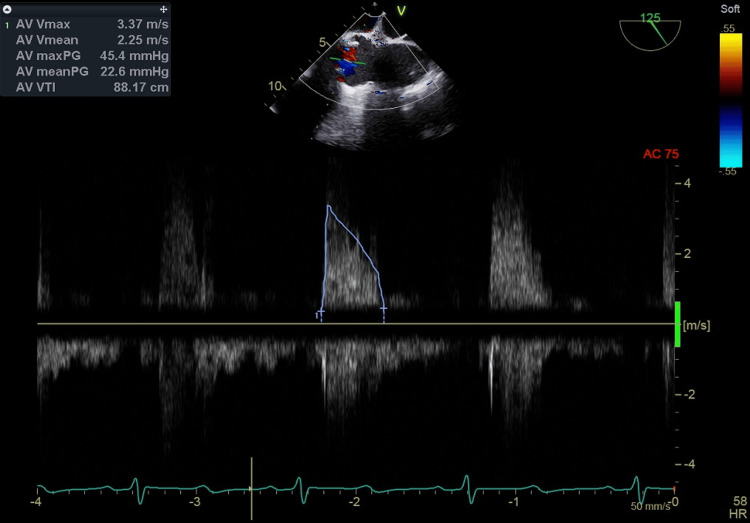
Assessment of blood flow velocity in accelerated blood flow corresponding to moderate AS by continuous wave Doppler of TEE after CPB It revealed a maximum aortic valve blood flow velocity of 3.4 m/s, a maximum aortic valve pressure gradient of 45 mmHg, and a mean aortic valve pressure gradient of 23 mmHg, indicating findings corresponding to moderate AS. AS: aortic stenosis; TEE: transesophageal echocardiography; CPB: cardiopulmonary bypass
